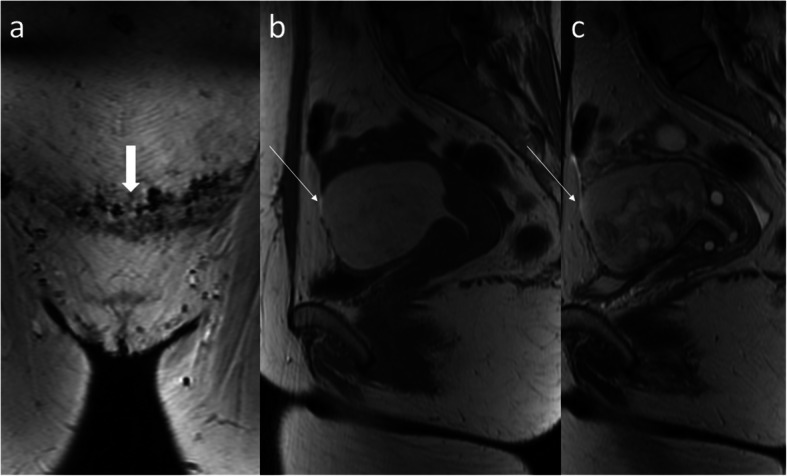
Pelvic MRI. A 42-year-old woman with a history of three C-sections. a GRE/T2*WI in the coronal plane (skin scar is shown by a thick arrow). b FSE/T1WI and c FSE/T2WI, both in the sagittal plane, depict a huge blood reservoir (T1-hyperintense, of mixed signal intensity on T2WI) with no serosal layer (no hypointense line) on the outer surface (thin white arrows). In pathology report: adenomyosis with autoamputation of the uterine body
